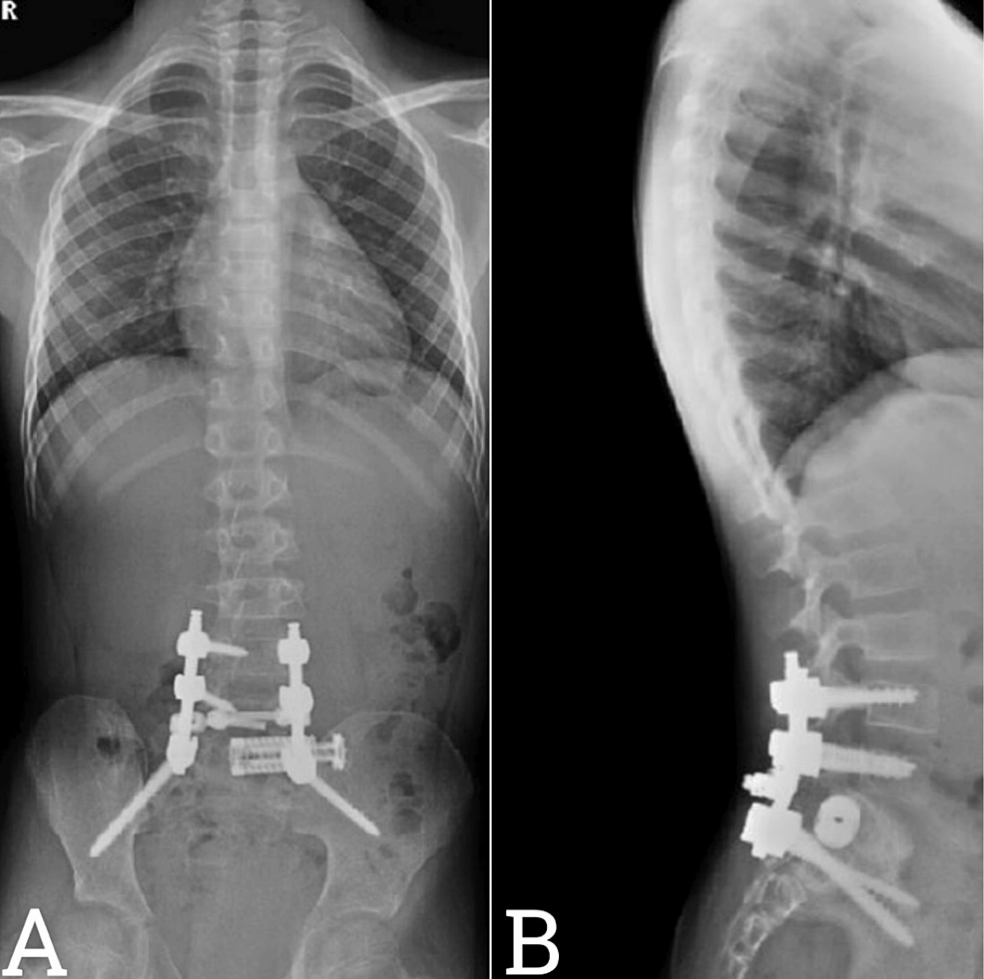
(A) Anteroposterior and (B) lateral whole spine X-rays show L4/5 instrumentation with bilateral S2 iliac screws with a horizontally placed expandable cage at the left L5 hemi corpectomy site to the left iliac crest
